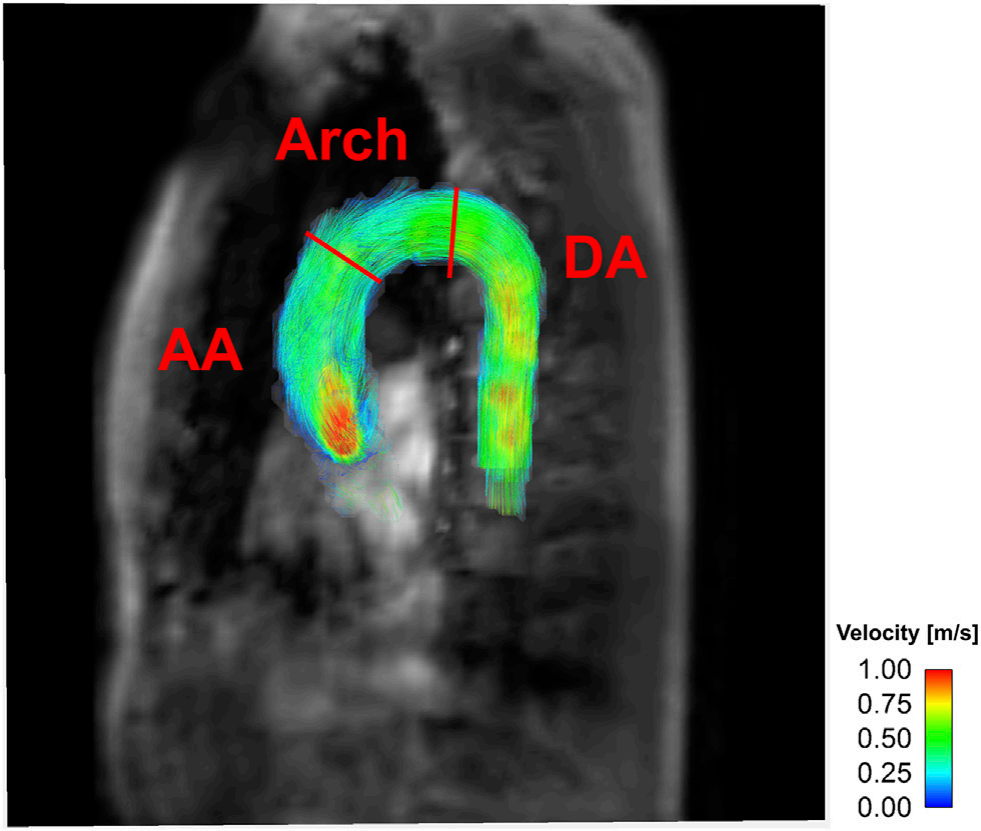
Representative velocity mapping of ICOSA6 4D flow MRI for *in-vivo*.
